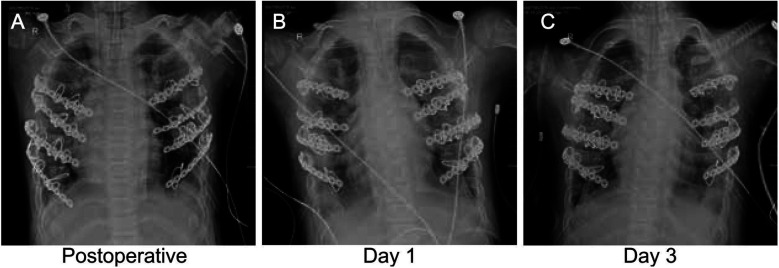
Continuous monitoring of chest x-ray: postoperative and early recovery periods. (A) Immediate postoperative chest x-ray: This image displays the immediate outcomes of thoracic correction surgery, showcasing the precise placement of thoracic stabilizers and closed chest drainage tubes. (B) Day 1 postoperative chest x-ray: The positioning of the chest wall stabilizer, alongside the placement of endotracheal intubation and central venous catheter, is visible in this image. (C) Day 3 postoperative chest x-ray: This image monitors the position of the chest wall stabilizer, evaluates the effectiveness of chest drainage tubes, and assesses the recovery of the lungs.
